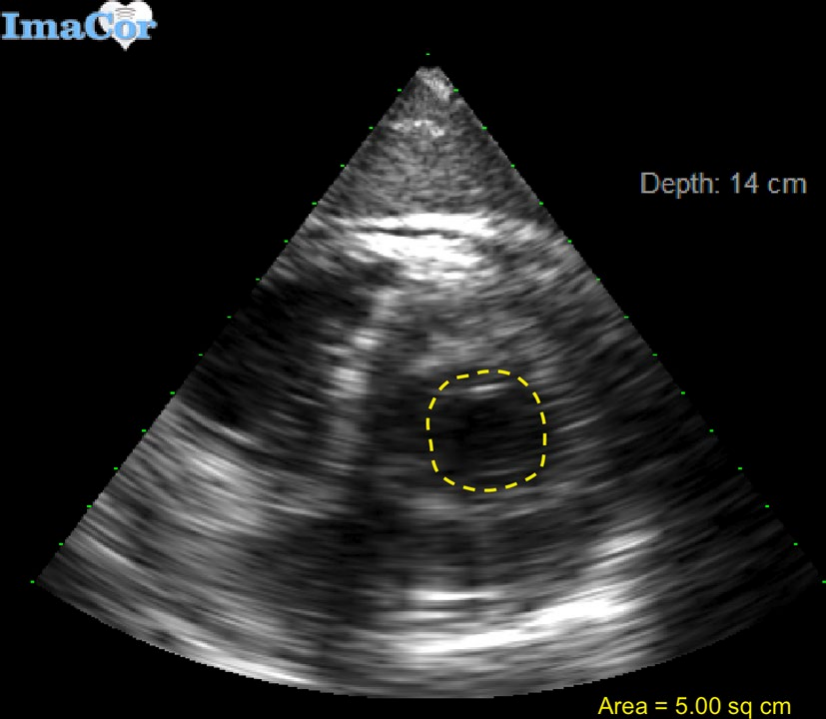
Transesophageal echocardiographic view demonstrating area tracing of left ventricle in end-systole
